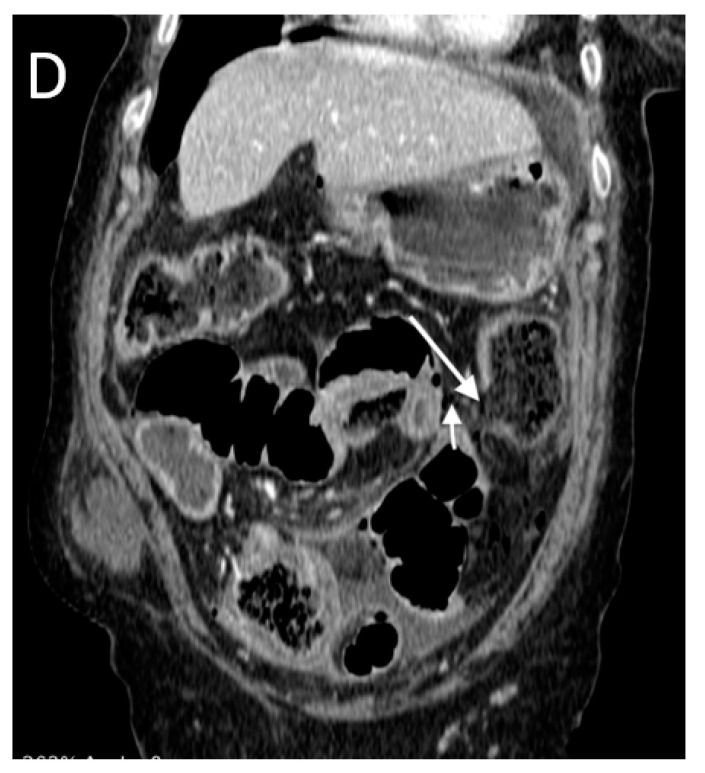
A 67-year-old female with a descending colon perforation. CT images show both supra- and inframesocolic free intraperitoneal air (arrows in (A,B)). A focal wall defect was identified (arrow in (C,D)). Note the air bubbles near the perforation site (short arrow in (D)) and the reactive segmental wall thickening with the submucosal edema of the ileal loop close to the colonic wall discontinuity.
